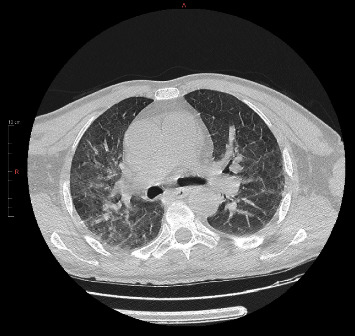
Chest CT showing ground-glass opacities and infiltrate of the right lung.
